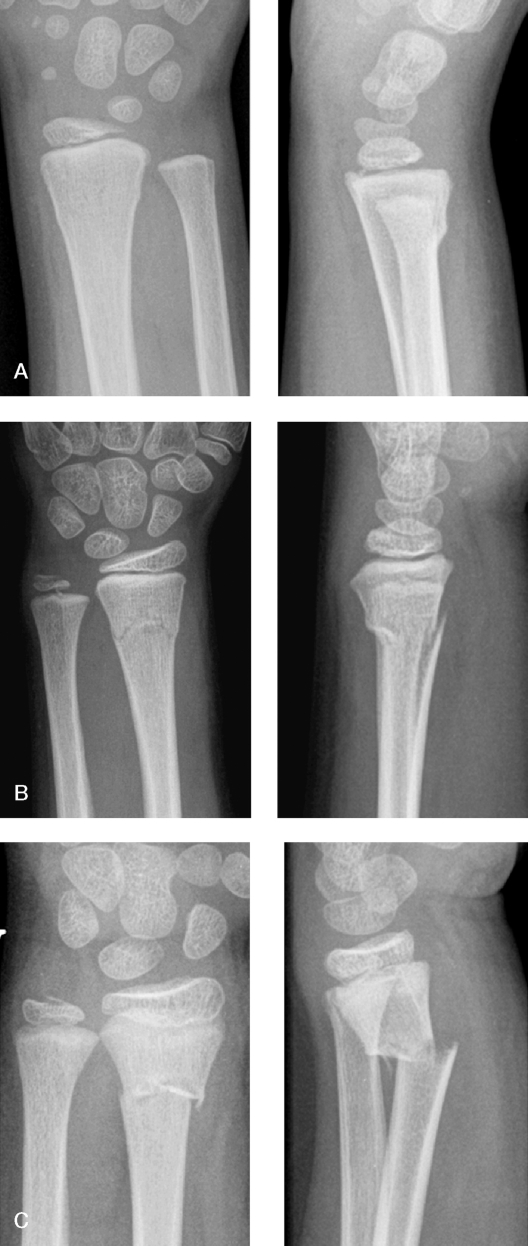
Primary radiographs of 3 different metaphyseal fractures, demonstrating the difference in cortical involvement in buckle (A), greenstick (B), and complete (C) fractures. A. Buckle fracture in a 7-year-old girl. B. Greenstick fracture in an 8-year-old girl. C. Complete fracture in a 7-year-old boy.
